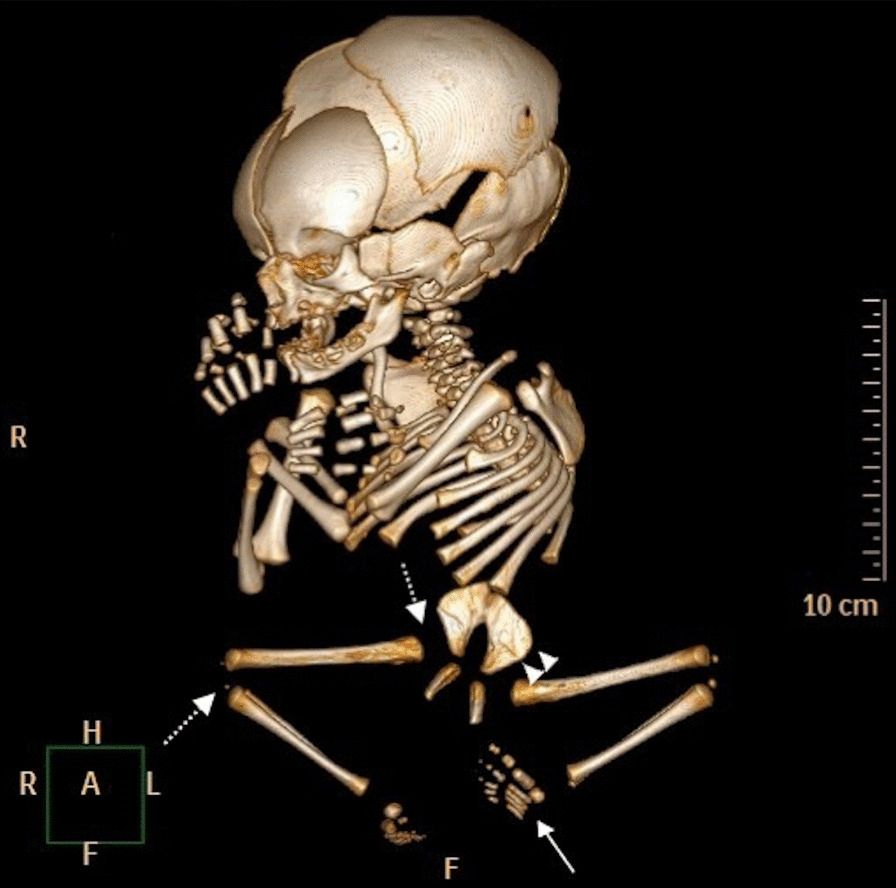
Postmortem computed tomography of the stillborn male. 3D image shows flexion and abduction at the hip with flexion at the knees (dotted arrows), left club foot (arrow), and hypoplastic iliac wings (arrow heads)
